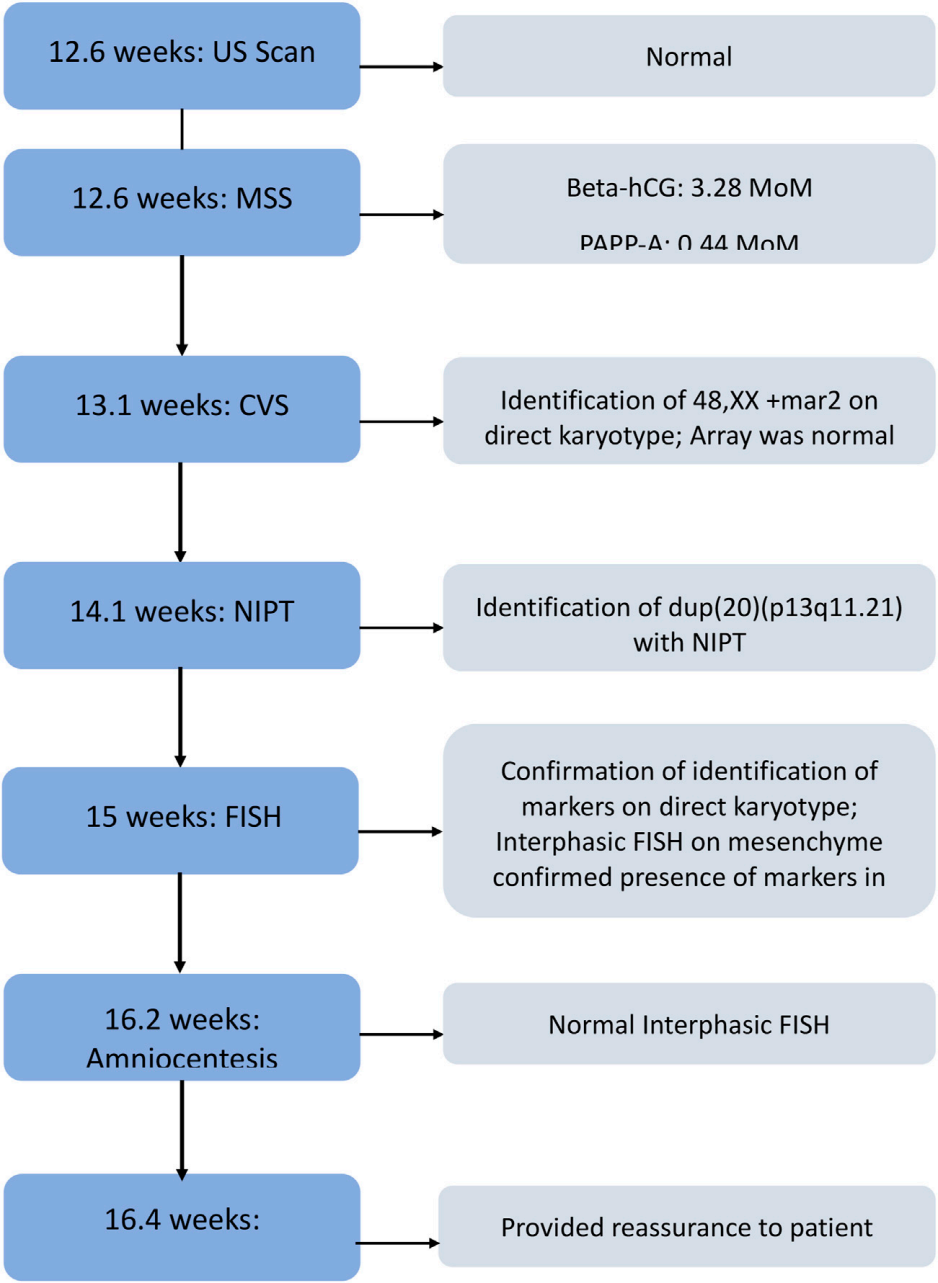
Patient’s analysis workflow by weeks of amenorrhea. US, ultrasound; MSS, maternal serum screen, CVS, chorionic villus sampling, NIPT, noninvasive prenatal testing; FISH, fluorescence *in situ* hybridization.
